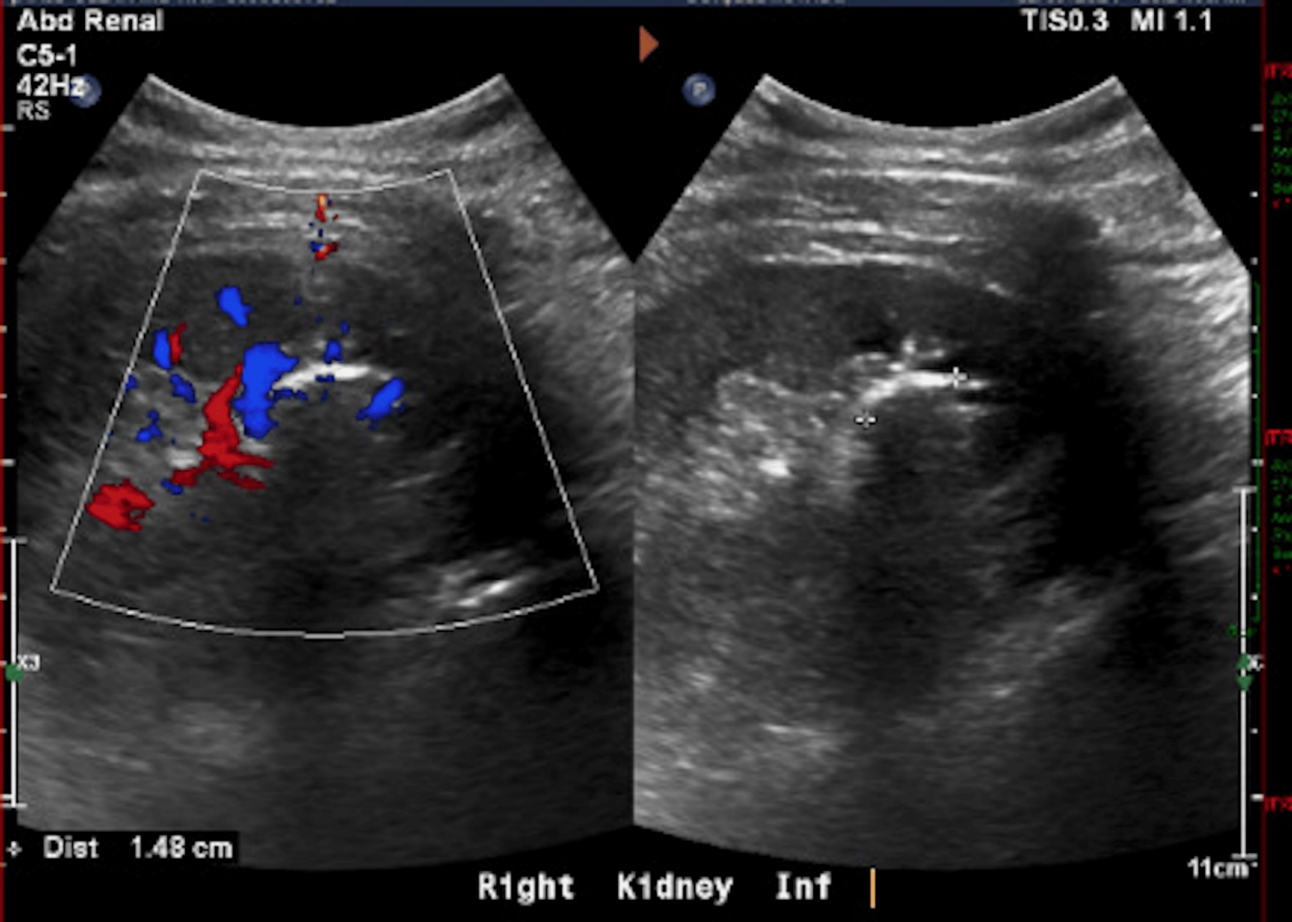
Repeat ultrasound of the right kidney showing a renal calculus measuring 1.4 cm
